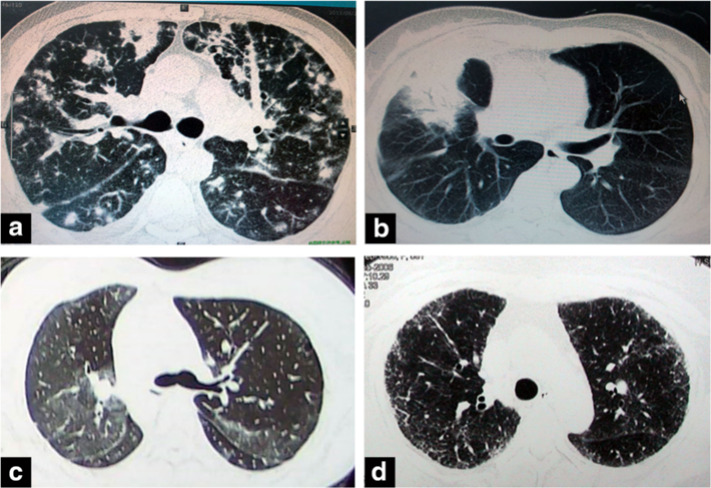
Different manifestations of IgG4-related lung disease via chest computed tomography (CT) scans. a Multiple nodules and masses with variable sizes are shown in both lungs via CT scans. Peribronchovascular thickening and thickened interlobular/interlobar septa were also noted. b Alveolar consolidations with air bronchograms in the right upper lobe. c Multiple ground glass opacities without reticular opacity. d Ground glass opacities with reticular opacities and early honeycombing
